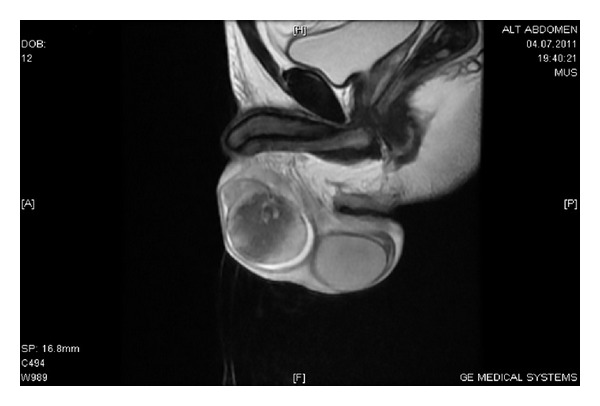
The scrotal MRI image of a patient with BEO.
